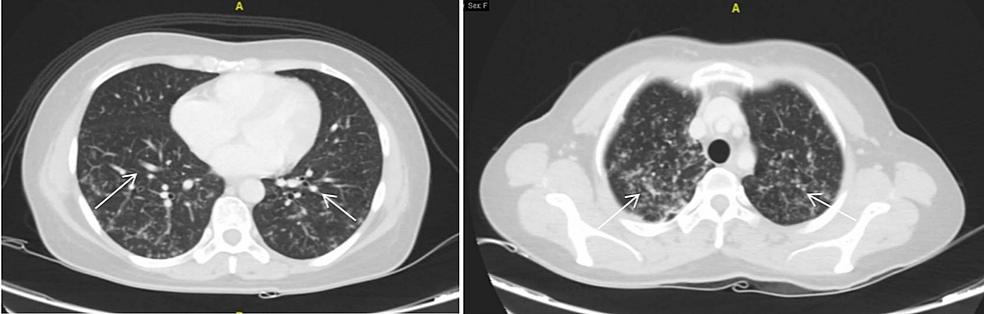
Axial CT images of the lung demonstrating diffuse tree-in-bud appearance with micro-nodularity (arrows) involving upper (left) and lower lobes (right). CT: computed tomography
